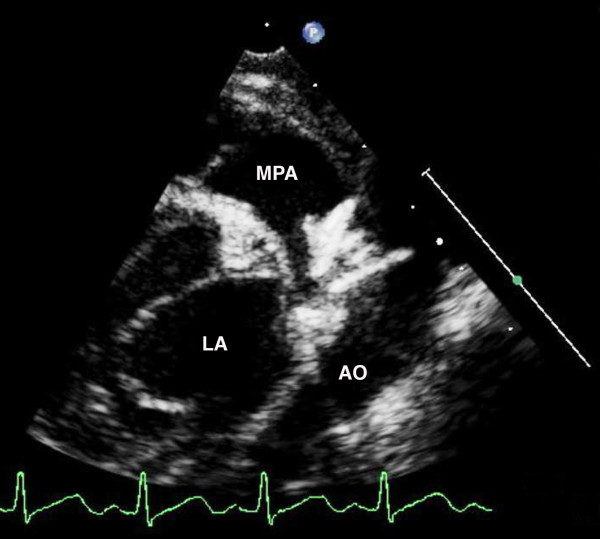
Echocardiogram following closure of patent duct with an Amplatzer ductal occluder II™. This image is taken from the same patient as figure 3c. This high parasternal long axis clearly shows the device fixed in the duct between left pulmonary artery and descending aorta.
